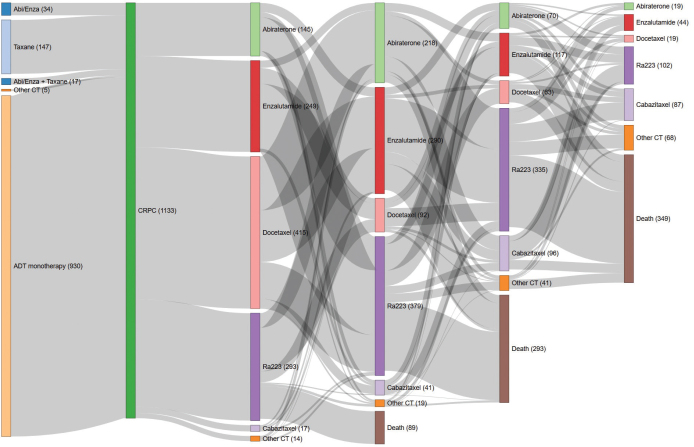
Line of sequence for radium-223-treated men with mCRPC in Sweden 2014–2020. Abi: Abiraterone; Enza: enzalutamide; Taxane: Docetaxel; CT: chemotherapy; ADT: androgen deprivation therapy; CRPC: castration-resistant prostate cancer; Ra-223: radium-223.
